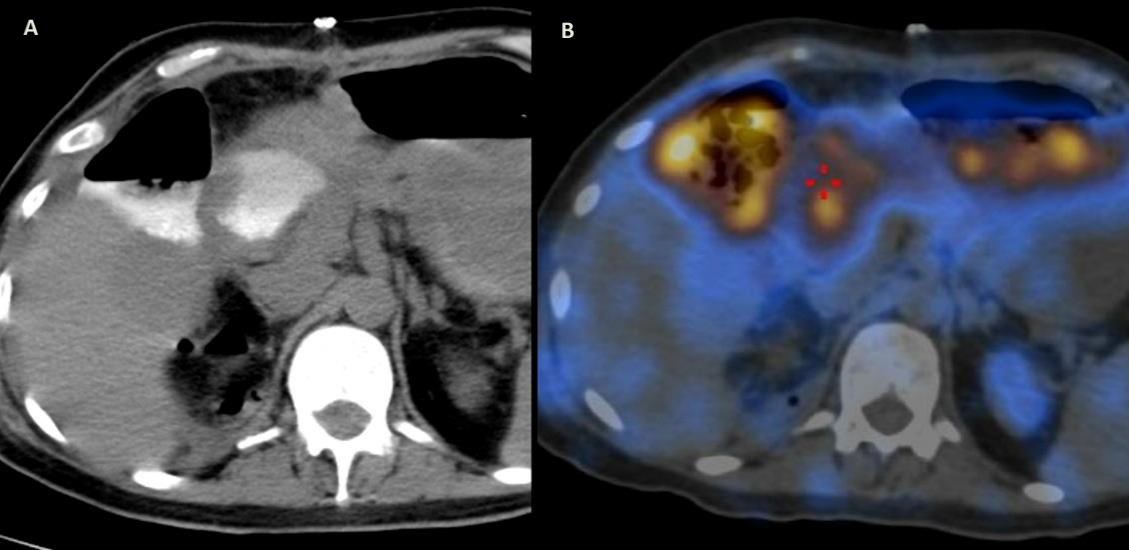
(A) CT image showing contrast in duodenum following contrast injection through percutaneous nephrostomy catheter (exact site of perforation was not delineable but was suspected to be through D2 segment of duodenum). (B) Fused image showing DTPA tracer uptake in pericolic space as well as duodenum and stomach
